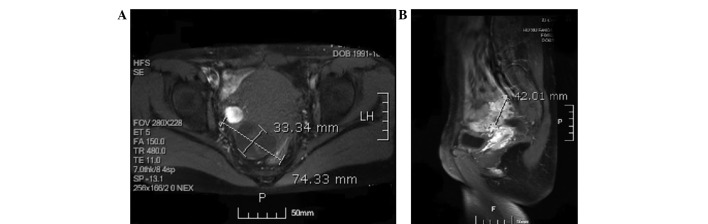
Magnetic resonance imaging revealed a cystic and solid mass in the right parametrium, measuring 3.3×7.4×4.2 cm. The tumor diameter was (A) 3.3cm×7.4 cm in the transverse plane and (B) 4.2cm in the coronal plane.
